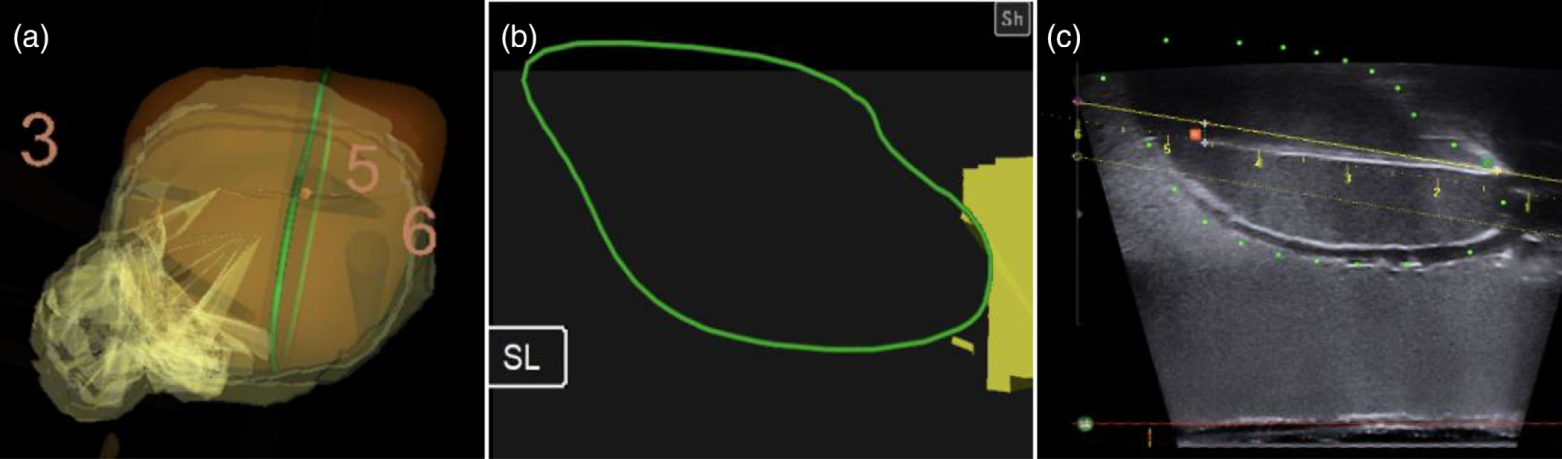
Enlarged view of targeting with previously bent needle for point 5. (a) 3D‐rendered uterus (orange) and CTV_HR_ (yellow) targeting point 5, with points 3 and 6 also in view; (b) contour of CTV_HR_ (solid yellow) and uterus (green) in the plane of the TRUS transducer when rotated to the plane of actual needle insertion. No intended needle track is shown, as the intended target was in a different plane; (c) live TRUS image in the plane of the TRUS transducer when rotated to the plane of actual needle insertion with points used for deviation measurement from the intended needle path (solid yellow) shown. TRUS, transrectal ultrasound.
